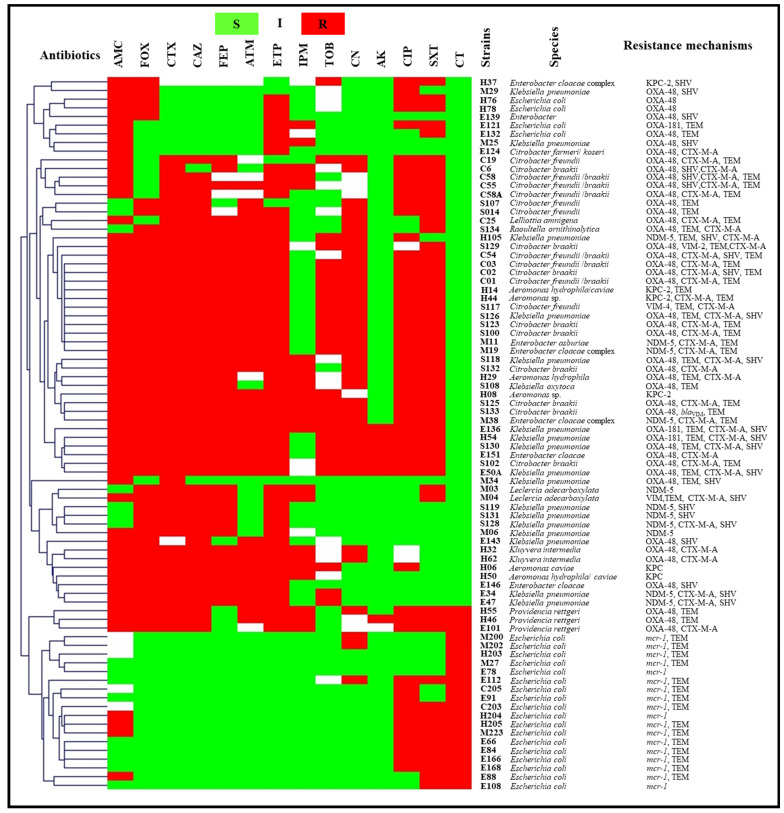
Antibiogram results and resistance mechanisms of enterobacteria and aeromonads isolates from wastewater clustered using the MultiExperimentViewer (MEV) software version 4_6_2. AK, amikacin; AMC, amoxicillin/clavulanate; ATM, aztreonam; CAZ, ceftazidime; CIP, ciprofloxacin; CN, gentamicin; CTX, cefotaxime; CT, colistin; ETP, ertapenem; FEP, cefepime; FOX, cefoxitin; I, intermediate; IPM, imipenem; PRL, piperacillin; R, resistant; S, susceptible; SXT, trimethoprim/ sulfamethoxazole; TIC, ticarcillin; TIM, ticarcillin/clavulanate; TOB, tobramycin; TPZ, piperacillin/tazobactam; Strains’ codes: C, cancer hospital; E, environment; H, university hospital; M, maternity hospital; S, public hospital.
